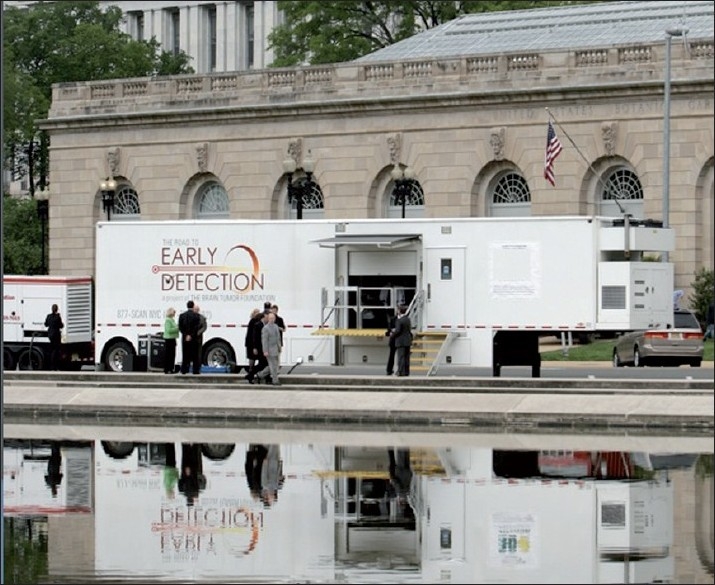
Mobile MRI unit at the reflecting pool in Washington, DC offering scans to members of the U.S Congress and their staffs. Results? Classified.
